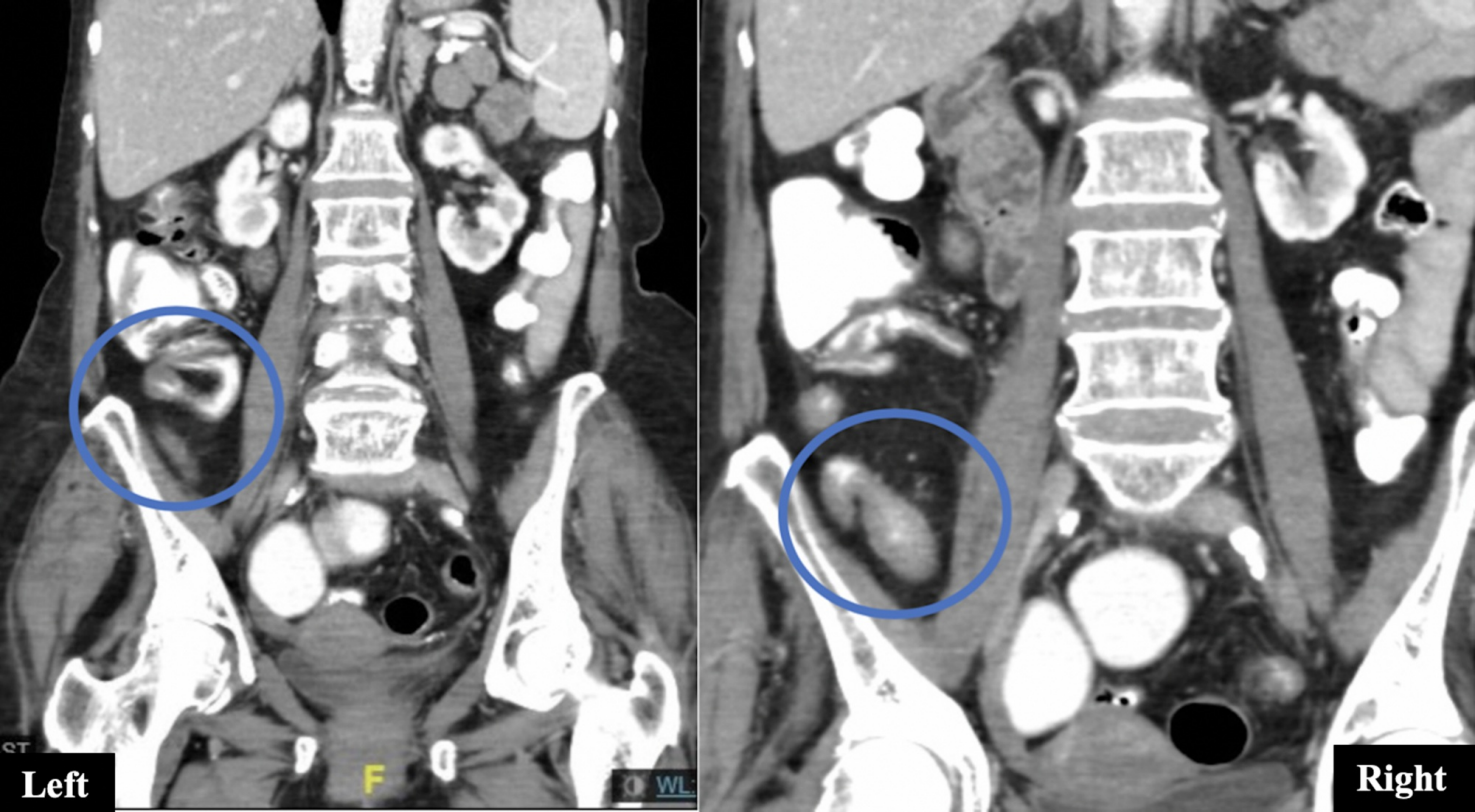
Left: CT scan on admission showing bowel wall thickening (circle). Right: CT scan post-corticosteroids therapy, showing worsening of wall thickening (circle; jejunal involvement is not shown). Computed tomography (CT)
